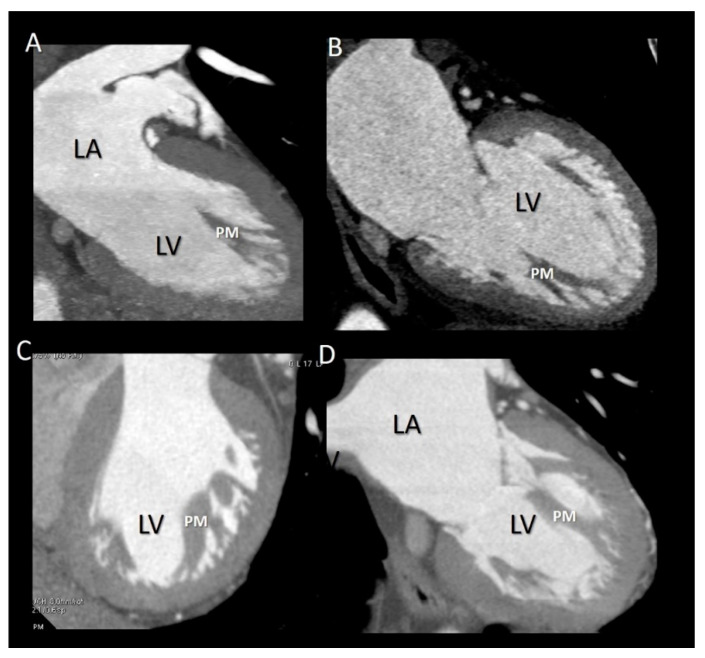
(A–D) CT representative images showing as the base of PMs do not attach to the compact myocardium but rather to a network of trabeculations.
